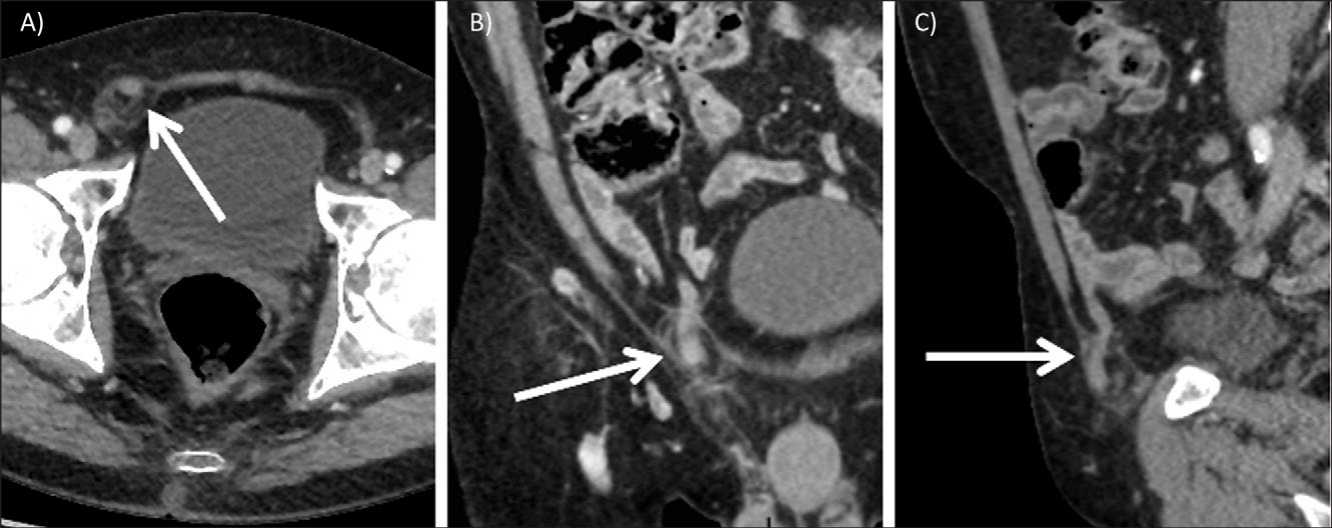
Contrast-enhanced computed tomography of the abdomen. Axial (A), coronal (B) and sagittal (C) views of the appendix located within the right inguinal hernia sac.
